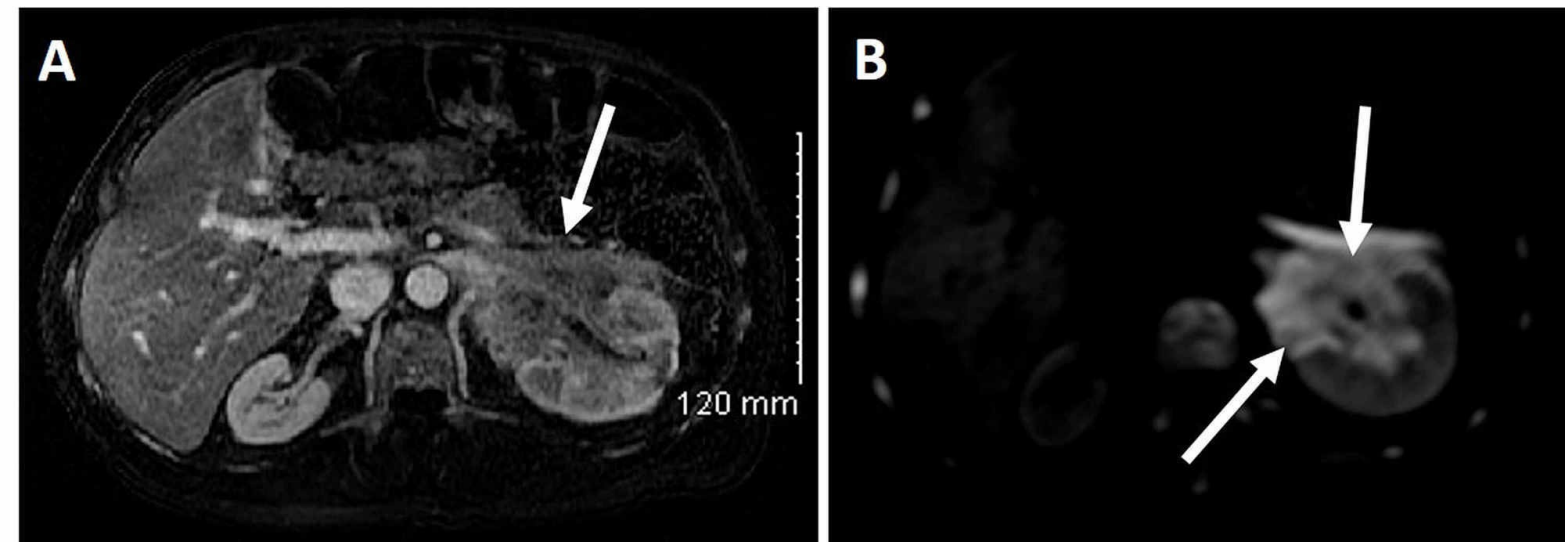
MRI of abdomen and pelvis. (A, B) A large ill-defined mass (arrows) was identified in the left kidney near hilum as visualized via T-1 and T-2 weighted images, respectively.
